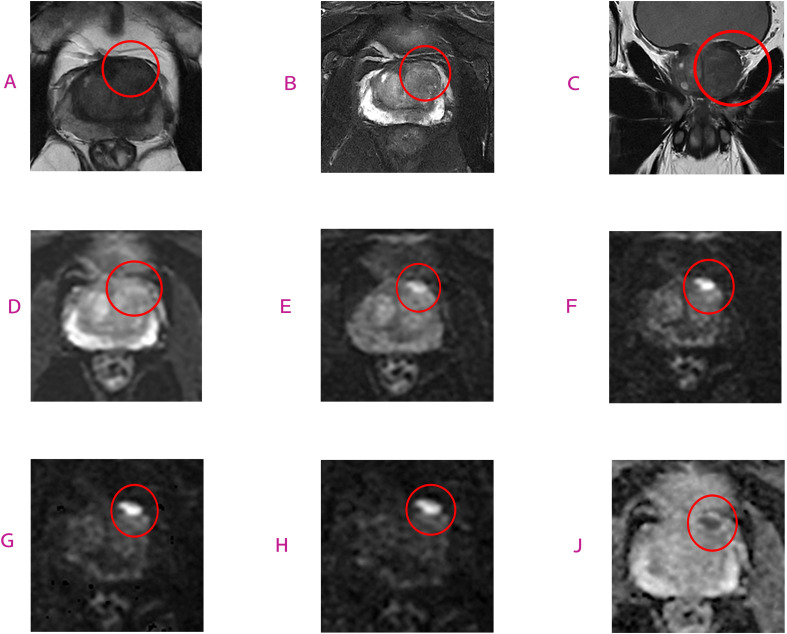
This is an MRI image of a 63-year-old patient with prostate cancer. T2-weighted images (A–C) show blurred lesion margins; DWI sequences at b=0 (D) and b=800 (E) are also shown; however, in the DWI sequences at b=1500 (F), b=2500 (G), and b=3000 (H), as well as the ADC map (J), the lesion (circled in the image) demonstrates distinct areas of diffusion restriction. Final pathological diagnosis confirmed transitional zone prostate cancer with a Gleason score of 9.
